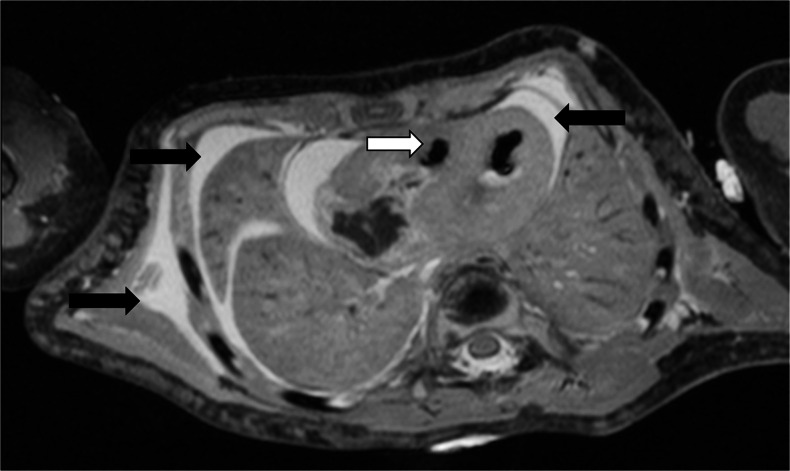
Fluid redistribution. Axial high-resolution T2-weighted postmortem magnetic resonance imaging in a term stillbirth. This demonstrates postmortem subcutaneous oedema, pleural and pericardial effusion (*black arrows*), and intracardiac gas (*white arrow*)
